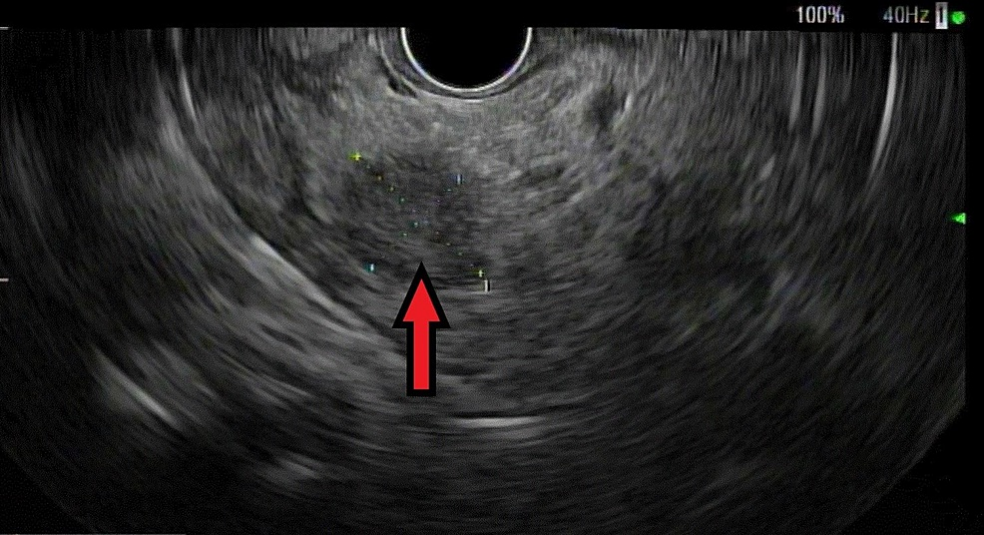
Endoscopic ultrasound image (red arrow) shows a 15 mm mass in the head of the pancreas with no vascular invasion or surrounding pathological lymph nodes.
